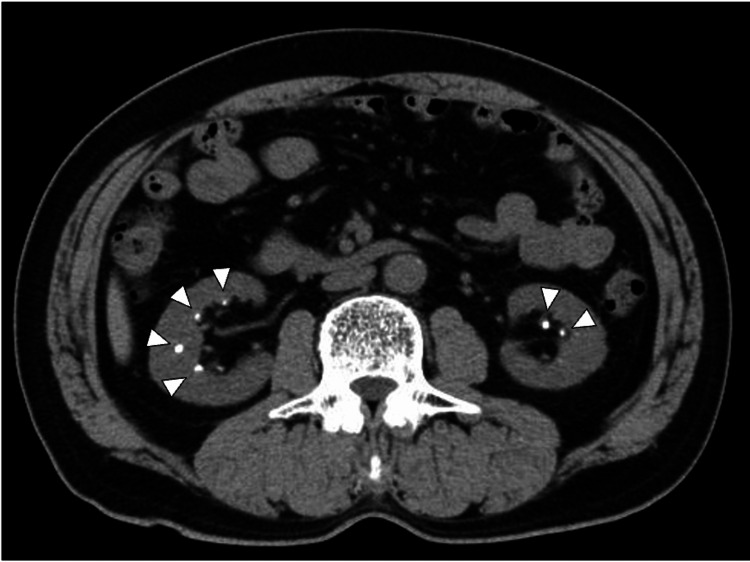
Abdominal computed tomography (CT) imaging showing bilateral renal calculi (arrows) without evidence of renal atrophy
